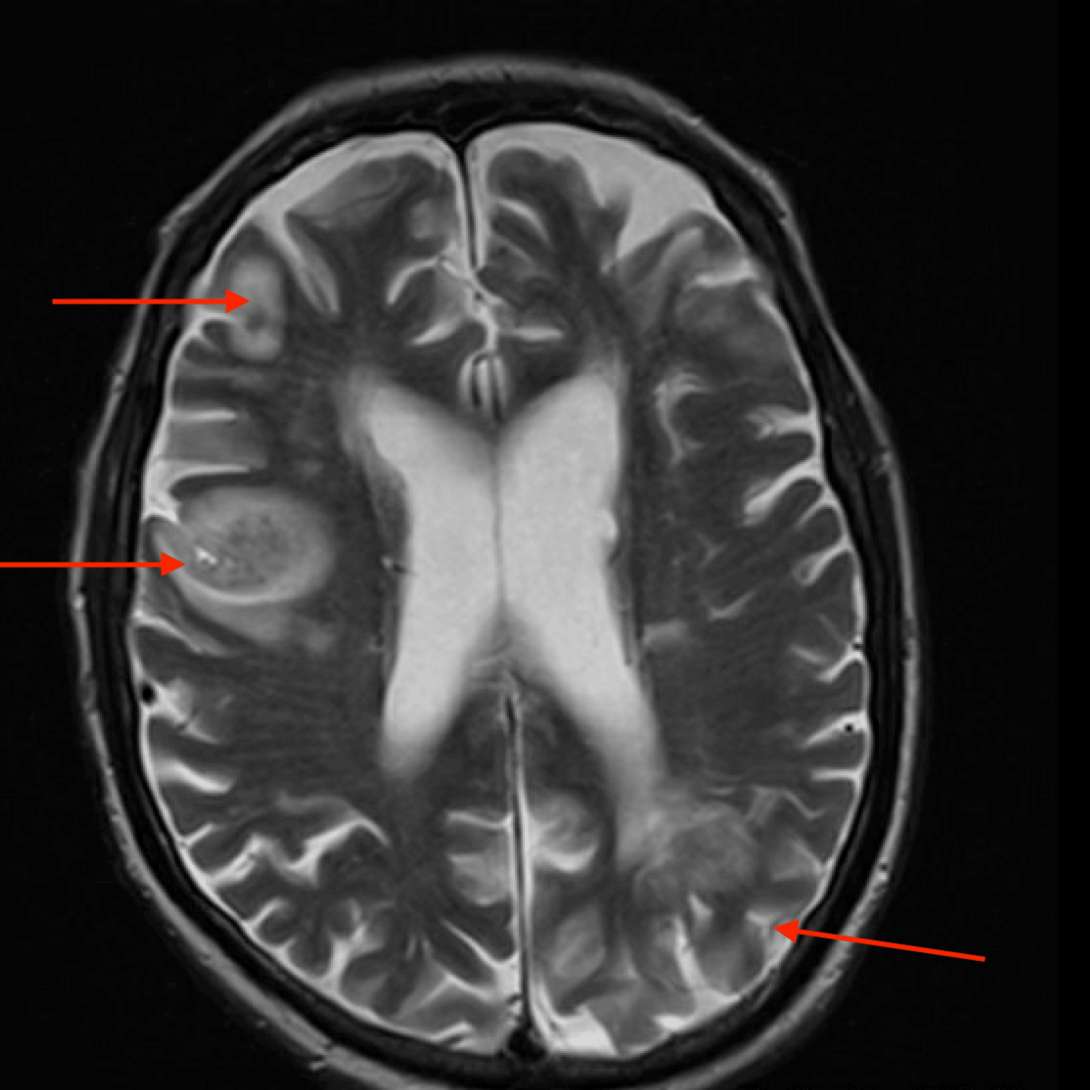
T2-weighted MRI brain showing multiple ischemic infarcts (red arrows)
